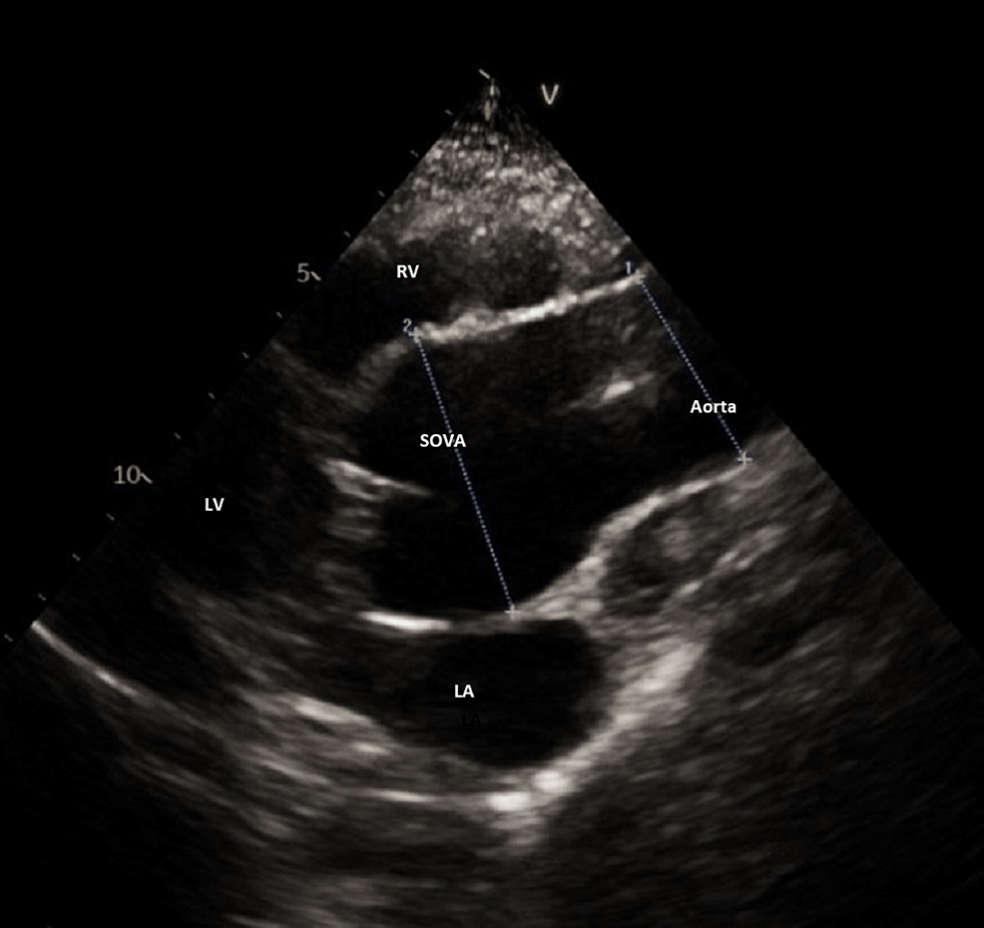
Echocardiogram revealing aneurysm of the sinus of Valsalva LA: Left Atrium; LV: Left Ventricle; RV: Right Ventricle; SOVA: SInus of Valsalva Aneurysm
